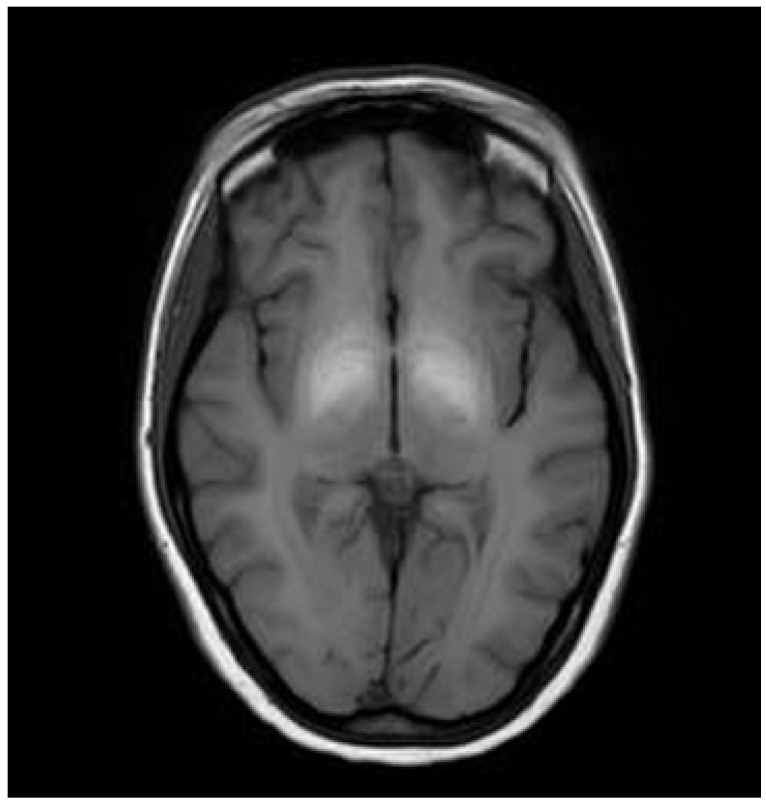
Hyperintense changes localized in both globii pallidi in T1-weighted images (probably due to manganese accumulation) (own materials of the neurology department).
